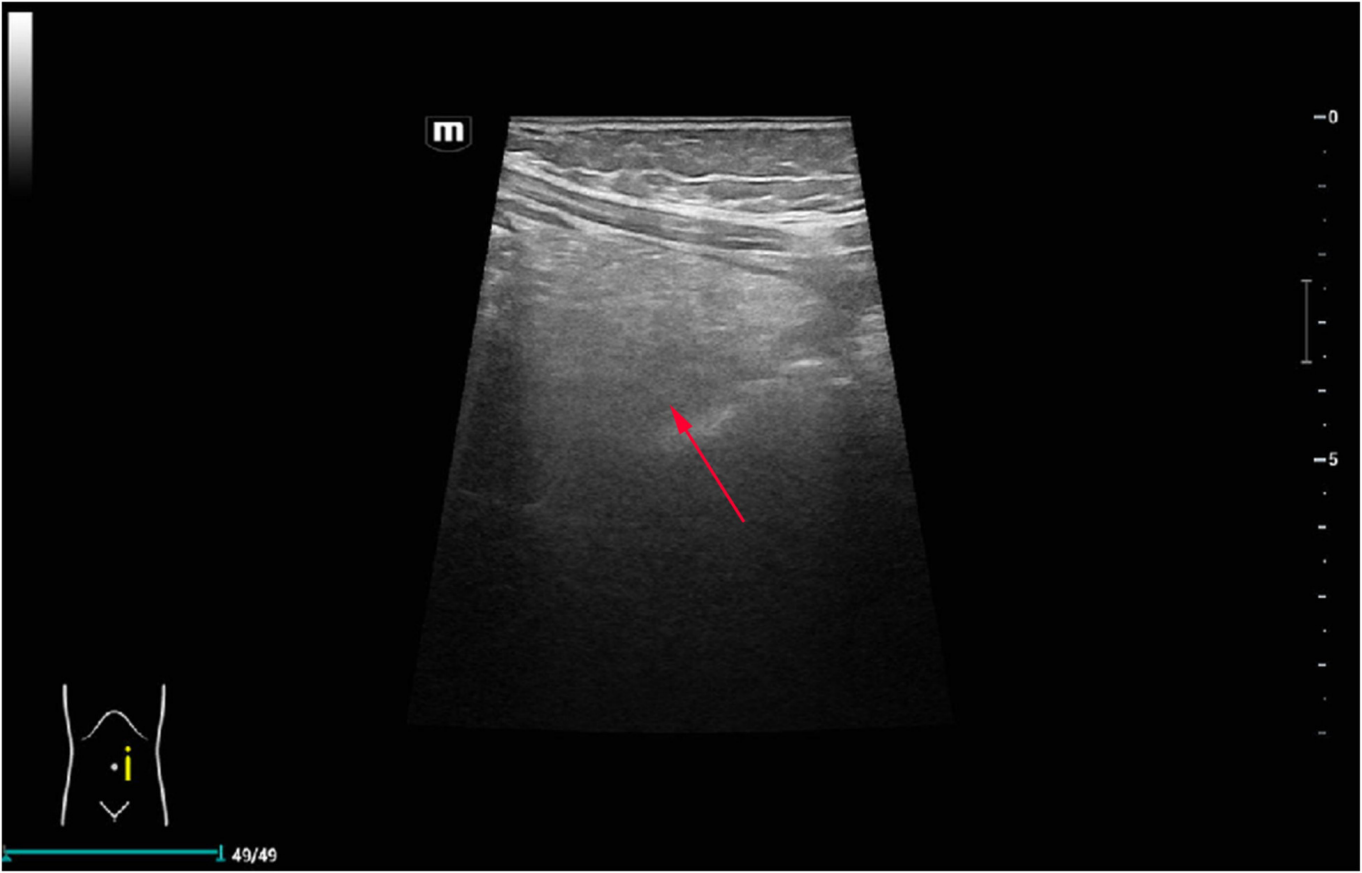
Postoperative follow-up Doppler ultrasound. The previously thickened left-sided omentum demonstrates restored internal blood flow (arrow) and more homogeneous echotexture, indicating resolution of the secondary torsion without infarction; persistent malrotation is noted without volvulus.
